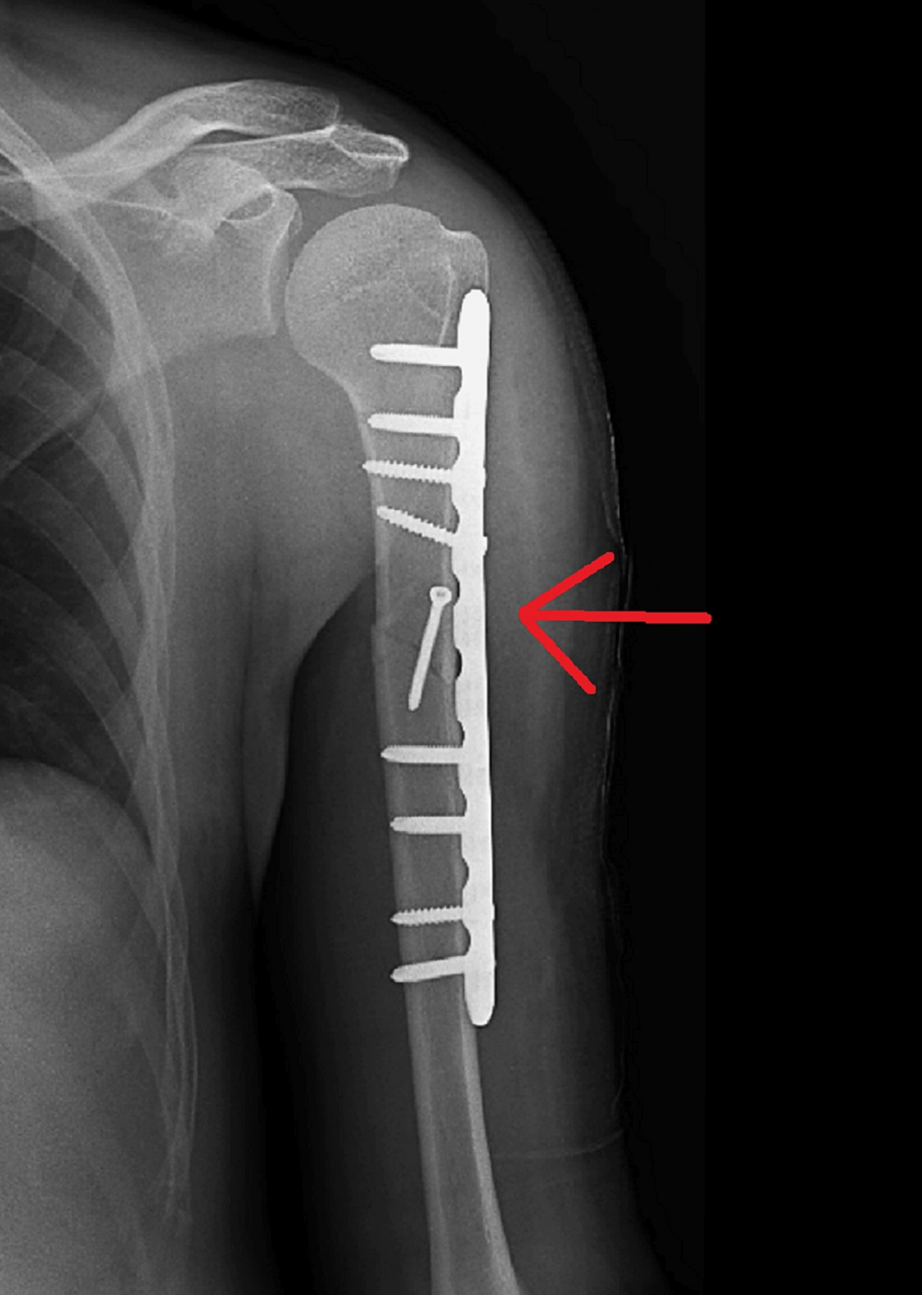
Anterior-posterior radiograph showing ORIF with plate osteosynthesis for proximal one-third humerus shaft fracture on left side ORIF - open reduction and internal fixation
